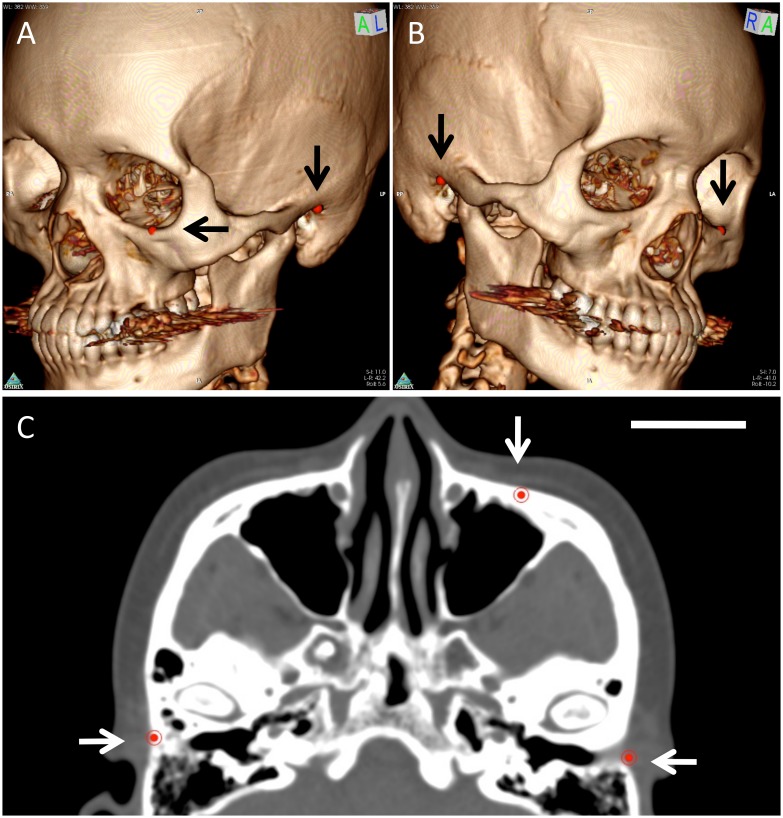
Frankfurt reorientation of the original CT DICOM data. After identifying the orbitale and porions on the 3D volume rendered skulls (black arrows, Fig. 1A and 1B), the Multiplanar Reconstruction function was used at 1mm slick thickness to identify the axial oriented slice that included the three defining points (white arrow) of the Frankfurt plane (Fig. 1C, scale bar = 3cm). The Multiplanar Reconstruction tool (not shown) can be used to identify any desired plane according to 3D landmarks using this function.
